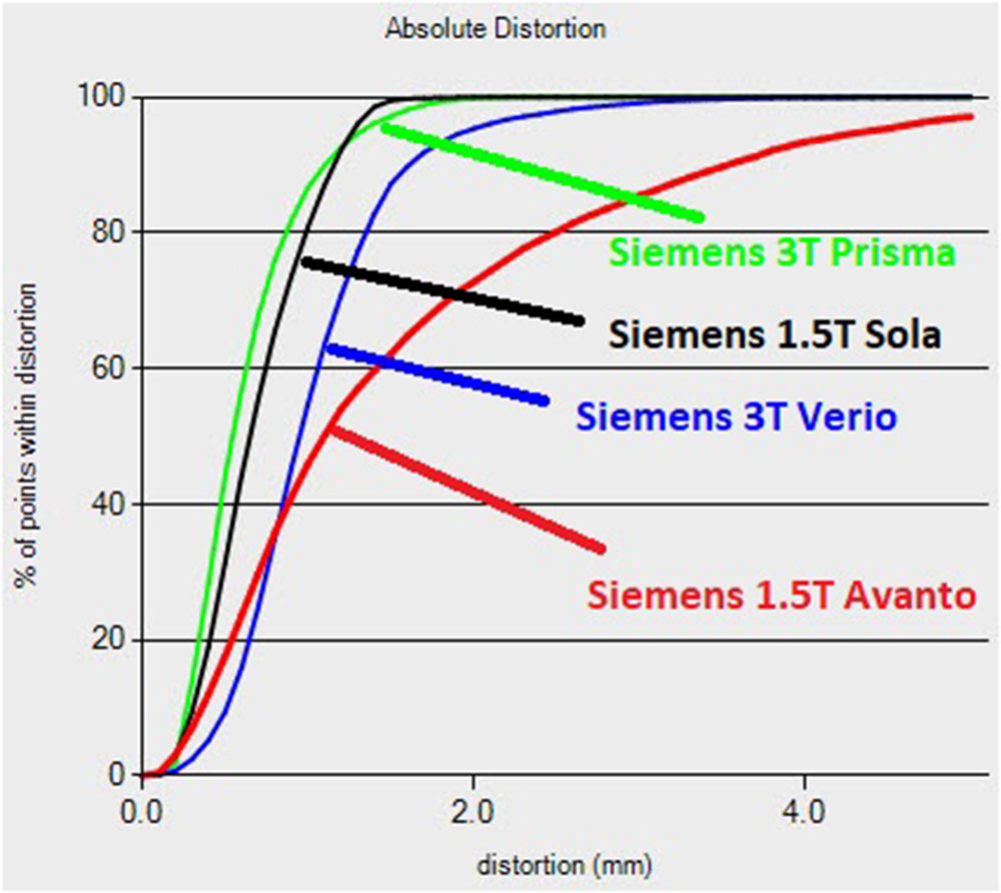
The problematic MRI machine Siemens 1.5T Avanto with large image distortion and the comparison with other scanners
